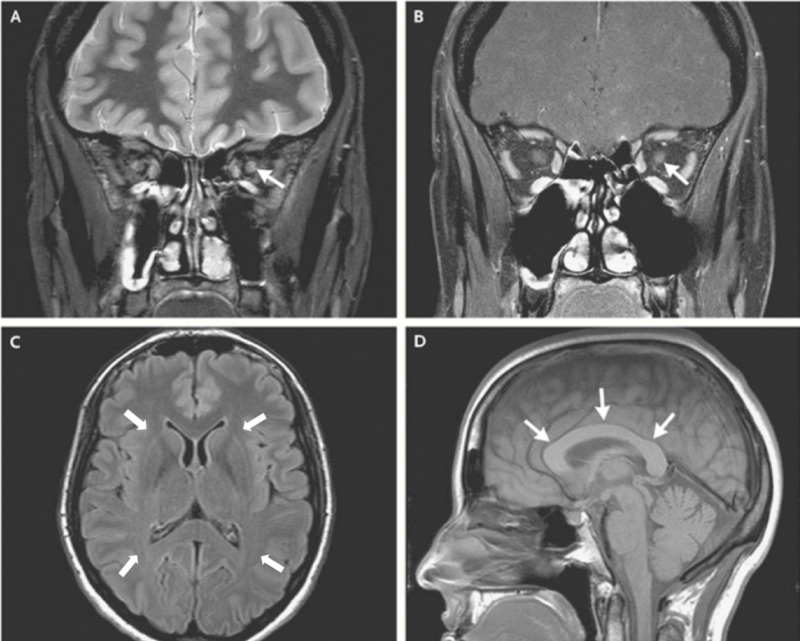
MRI of the Orbits and the Head. (A) Coronal, T2-weighted, high-resolution, short-tau-inversion-recovery MRI indicating an increased signal in the left optic nerve (white arrow). (B) Coronal, contrast-enhanced, high-resolution, fat-suppressed, T1-weighted image indicating mild hazy-enhancement of the left optic nerve (white arrow). (C) Axial, fluid-attenuated, inversion recovery imaging indicates no abnormal lesions of the white matter. (D) Sagittal, nonconstrast-enhanced, T1-weighted image indicating no lesions in the corpus callosum, which is normal in caliber and shape (white arrows). MRI, magnetic resonance imaging
